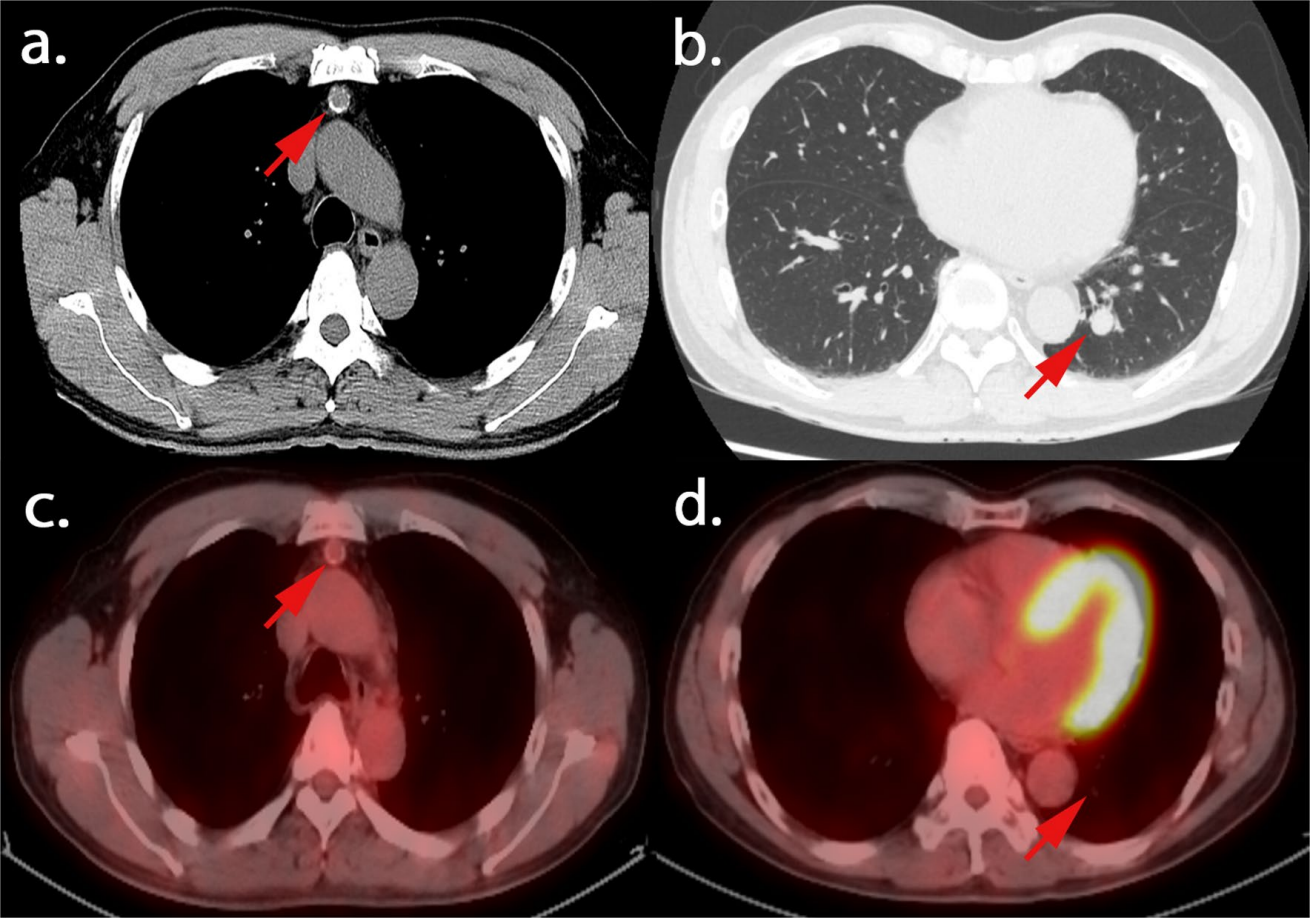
a, b Chest computed tomography findings. A tumor with calcification is located in the anterior mediastinum (a) and a well-defined hilar nodule is located in S^10^ of the left lung (b). c, d
